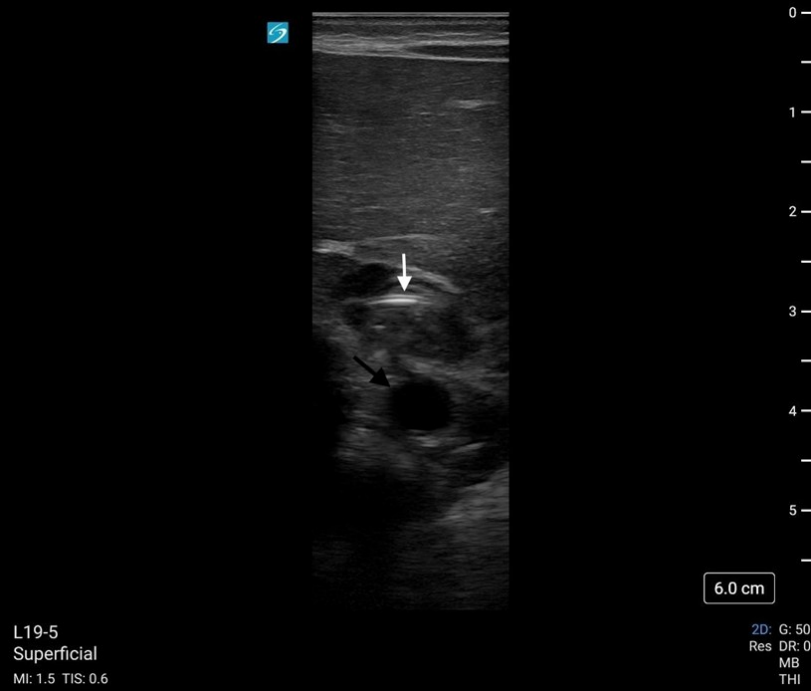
Enteral feeding tube (white arrow) visualized in esophagus using point of care ultrasound (POCUS). The aorta (black arrow) can be seen posterior to the esophagus and is used as a landmark.
